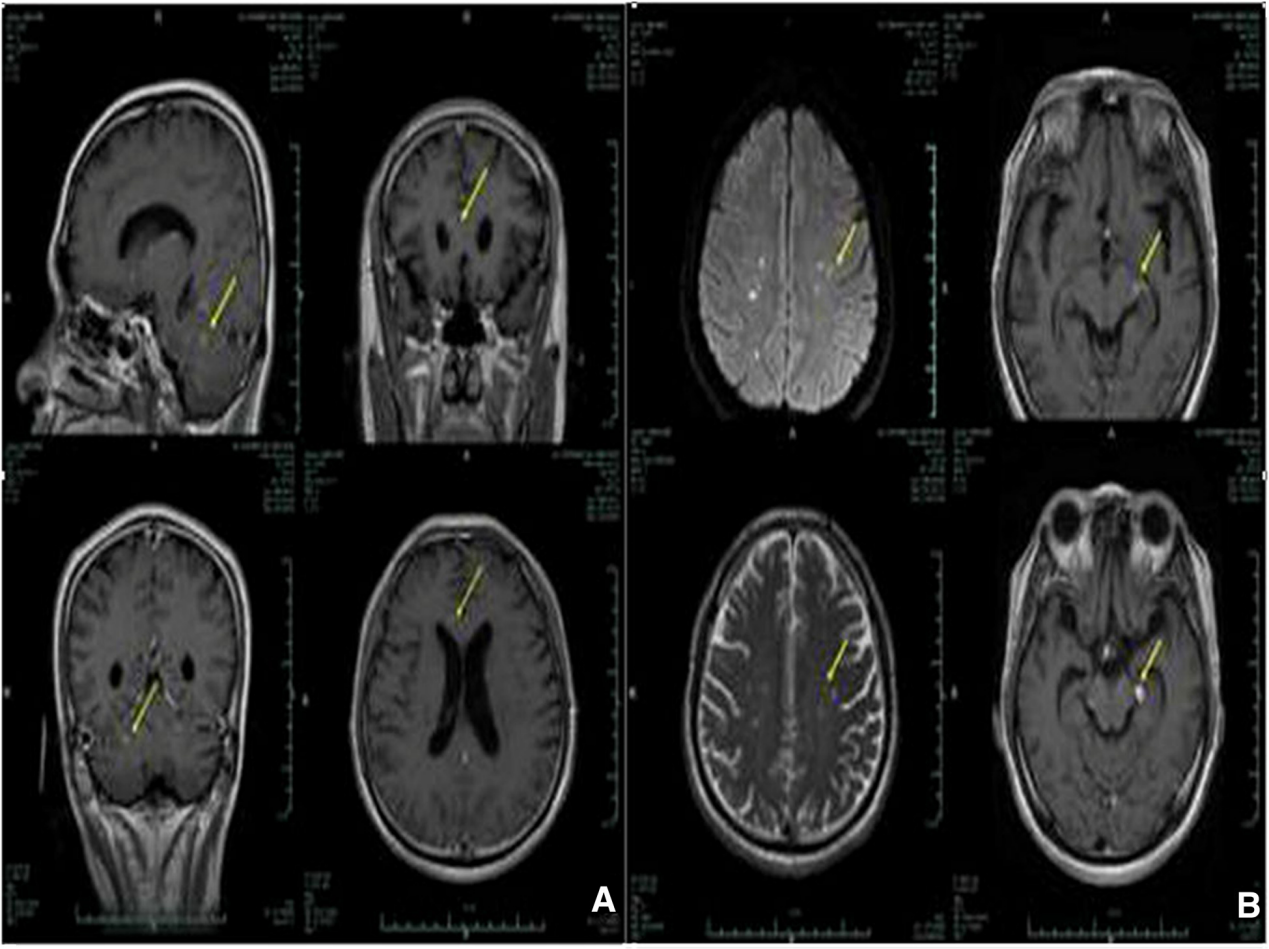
Head MRI. A. Head MRI taken on February 24; B. Head MRI taken on March 13. Comparison of the results of the head MRIs revealed the continued presence of meningeal infiltration and no obvious improvement in the parenchymal nodules in the brain.
